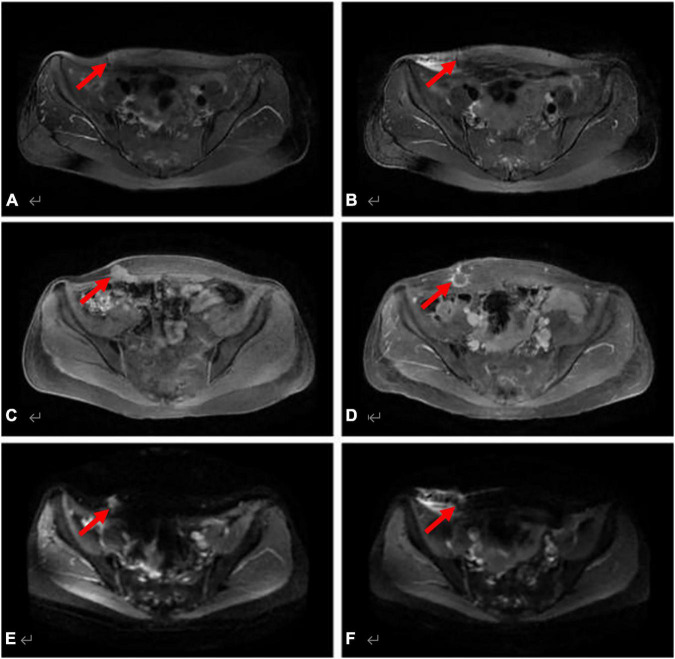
DWI sequence for a 35-year-old patient before and after HIFU treatment. The AWE lesion located at the right corner site of the surgical scar. This chart shows a comparison between pre- and post-treatment. (A) Axial T2WI with fat-suppression shows that the lesion appeared slightly hyper-intense (red arrow) pre-operatively. (B) Axial T2WI shows the same lesion (red arrow) appeared as signal reduction post-ablation, and the high signal in the surrounding tissue represents edema. (C,D) Axial contrast imaging showed evident enhancement pre-treatment (red arrow) and revealed non-enhancing areas of post-treatment (red arrow). (E,F) On DWI, the lesion showed high signal pre-operatively, the ADC value was 1.7 × 10^–3^mm^2^/s (*b* = 600 s/mm^2^), which showed slightly low signal post-treatment, and the ADC value was 2.7 × 10^–3^mm^2^/s (*b* = 600 s/mm^2^).
